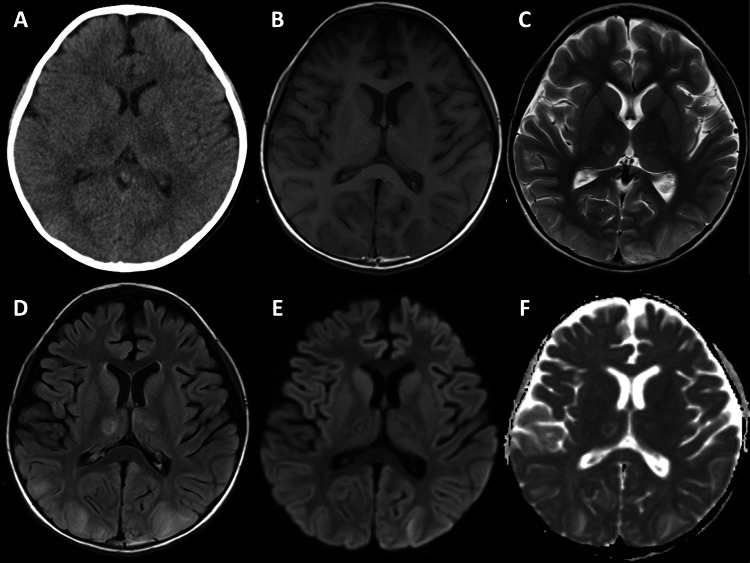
Imaging studies (A) day 2 CT scan, (B) day 16 T1-weighted image, (C) day 16 T2-weighted image, (D) day 16 fluid-attenuated inversion recovery, (E) day 16 diffusion-weighted image, (F) day 16 apparent diffusion coefficient map
